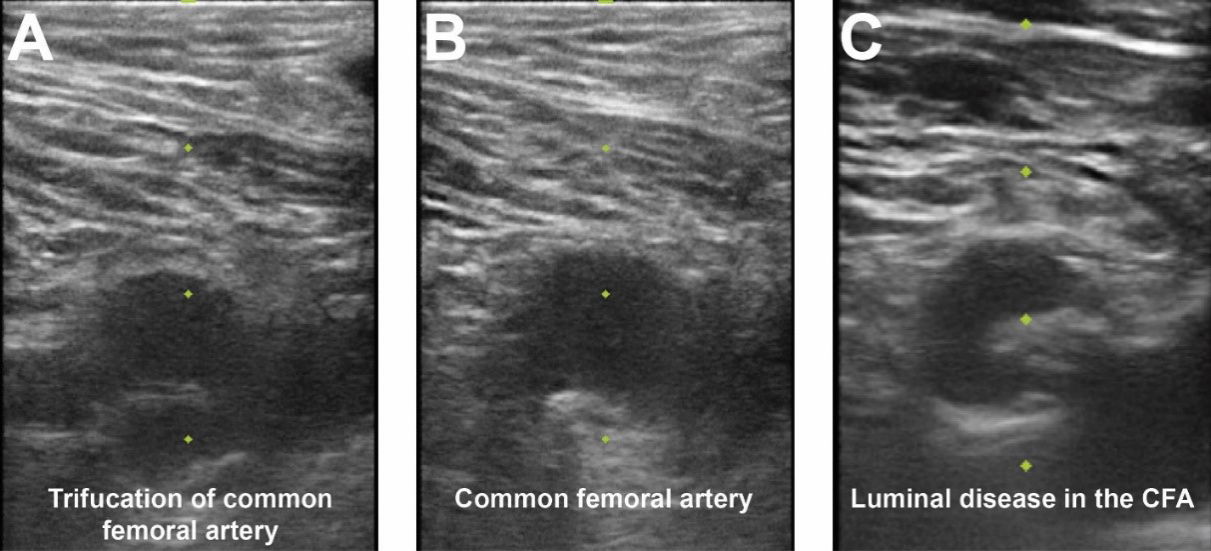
Femoral access ultrasound. (A) Femoral artery
